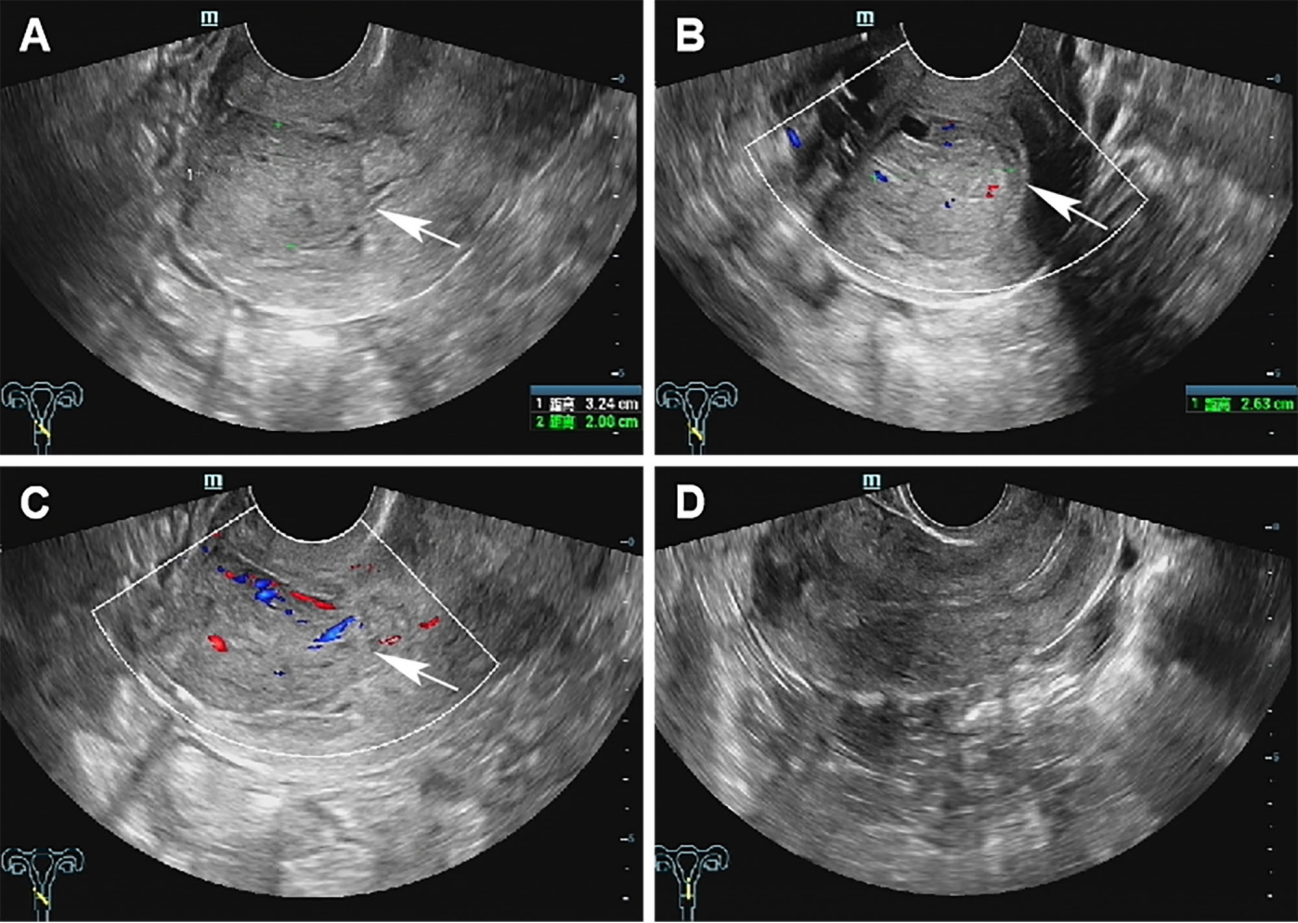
Preoperative and postoperative ultrasound images. (A) Transvaginal ultrasound longitudinal view revealed a medium-to-high echoic mass on the posterior lip of the cervix (white arrow). (B) Transvaginal ultrasound shows a transverse section image of the cervical mass (white arrow). (C) Color doppler flow imaging (CDFI) showed the cervical mass with slightly abundant blood flow signals (white arrow). (D) Transvaginal ultrasound revealed a normal uterus following adenomyomectomy.
